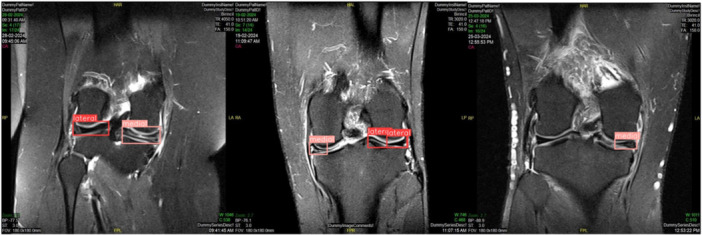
True positive (TP), false positive (FP) and false negative (FN) results in the frontal view of magnetic resonance imaging scans for meniscus tear detection using the YOLOv8 and EfficientNetV2 model.
